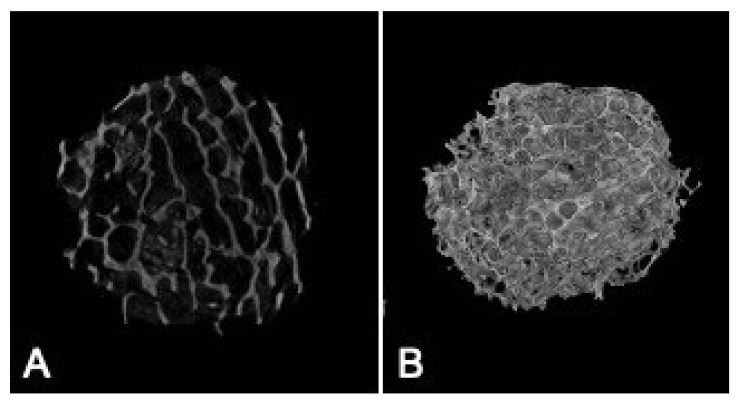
Representative μ-CT images of the CBHA scaffold. (A) The 2D cross section of the BHA scaffold; (B) The 3D rendering of the CBHA scaffold.
